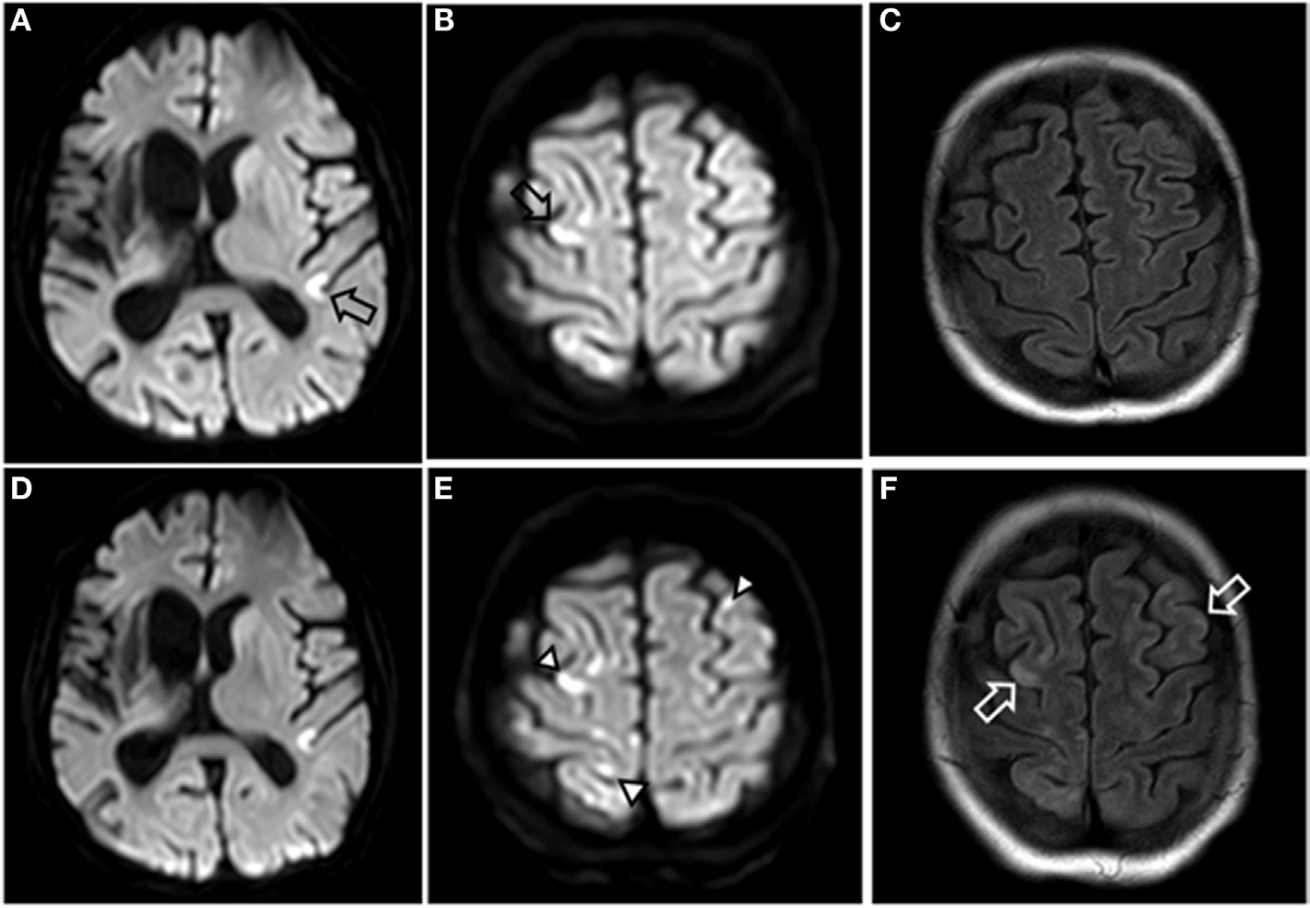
MRI images of brain. Diffusion-weighted imaging (DWI) 5 h after symptom onset showing multiple small hyperintensities in both hemispheres (open black arrows) (A,B), and fluid-attenuated inversion recovery (FLAIR) showed no lesions (C). Four days after onset, the multiple hyperintensities in DWI are better demarcated (closed black arrowheads) (D,E), and FLAIR is showing multiple hyperintensities consistent with small areas of brain injury (open white arrows) (F).
